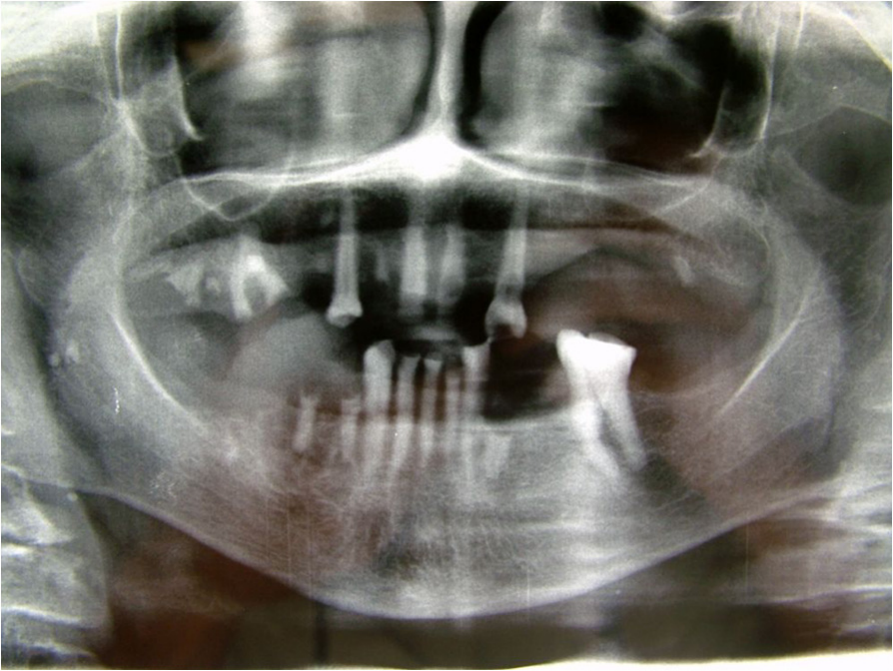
Orthopantomography, Patient 1
